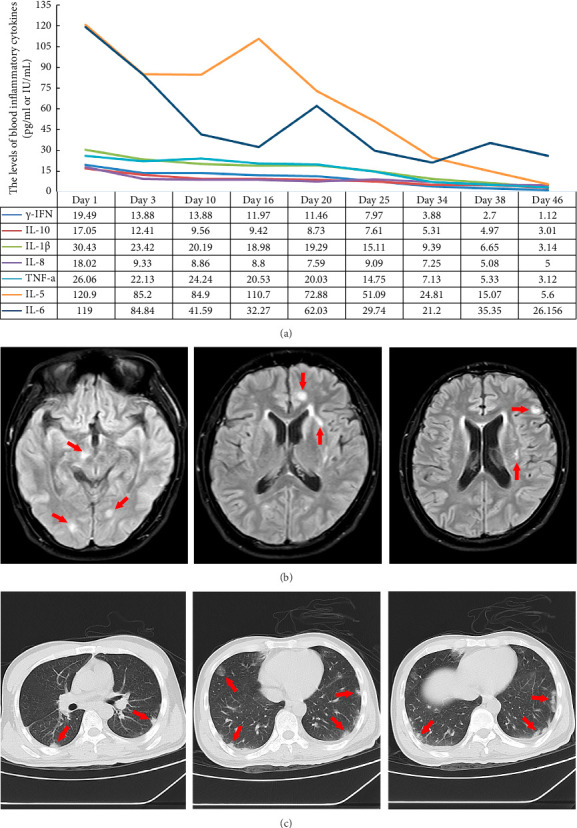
(a) The levels of inflammatory cytokines (γ-IFN, IL-10, IL-1β, IL-8, IL-5, IL-6, and TNF-α) exhibit a decreasing trend, particularly for IL-5 and IL-6. The unit of γ-IFN is international units per milliliter (IU/mL). The units of IL-10, IL-1β, IL-8, IL-5, IL-6, and TNF-α are picograms per milliliter (pg/mL). (b) The cranial magnetic resonance imaging scan showed subcortical abnormalities in both cerebral hemispheres on the T2-FLAIR sequence. (c) The lung CT scan showed several distinct inflammatory signal changes near the pleura.
